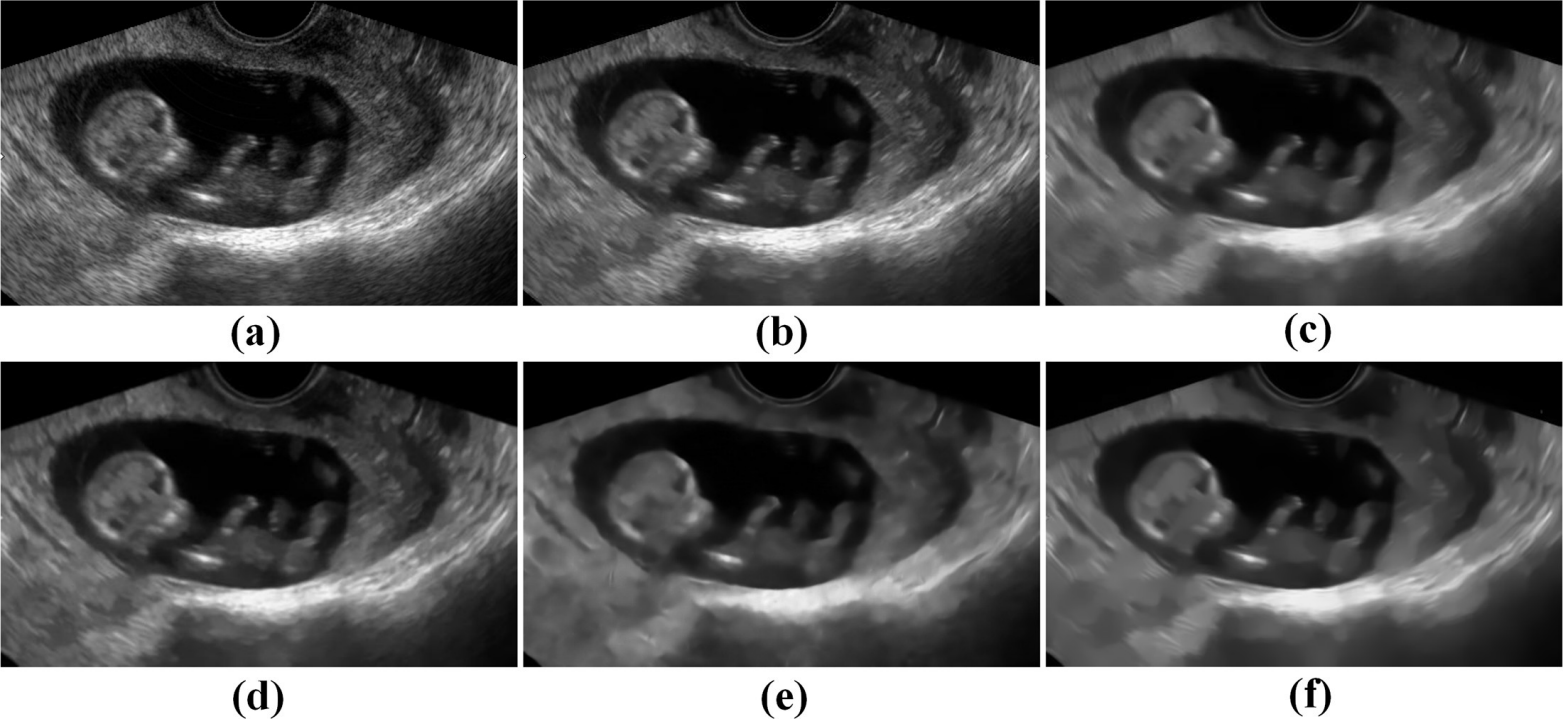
Comparison of restoration performance on a real fetal ultrasound image for the NLM-based and DnCNN methods. (a) original image, (b) TNLM filter, (c) OBNLM filter, (d) NLMLS filter, (e) DnCNN filter, and (f) PPCA-NLM filter.
